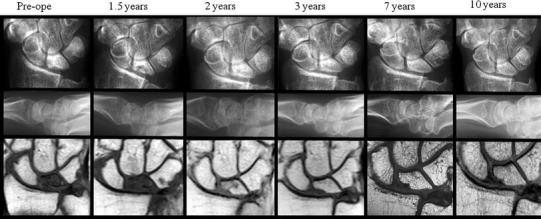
Roentgenograms and MRI for case 4. The images show that the lunate has not collapsed, and that the lunate intensity is recovered on MRI. The *upper panels* show anteroposterior views on the roentgenograms, the *middle panels* show lateral views on the roentgenograms, and the *lower panels* show PDW images on MRI. The preoperative images are shown on the *left*, together with the 1.5-, 2-, 3-, 7-, and 10-year postoperative images
